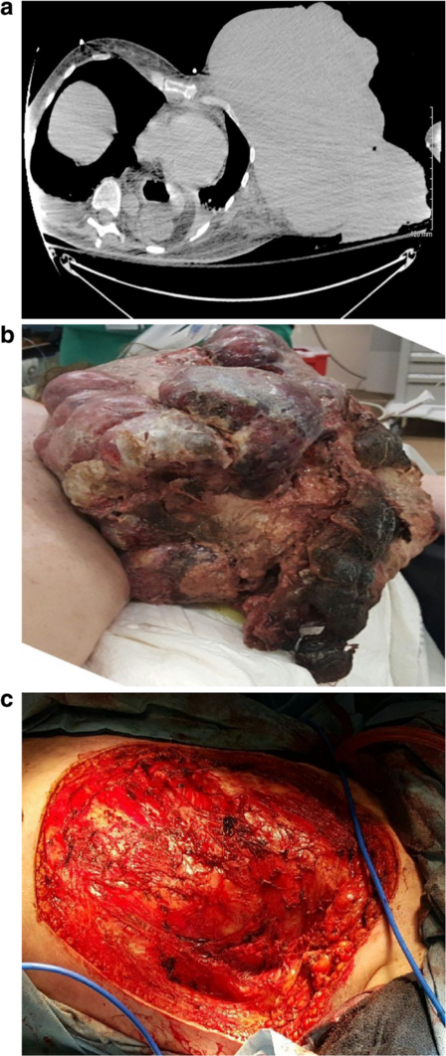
Gross appearance of the mass at presentation (a, *left panel*), computed tomography image without contrast of patient at presentation showing large exophytic mass (b, *center panel*), and postoperative appearance (c, *right panel*)
